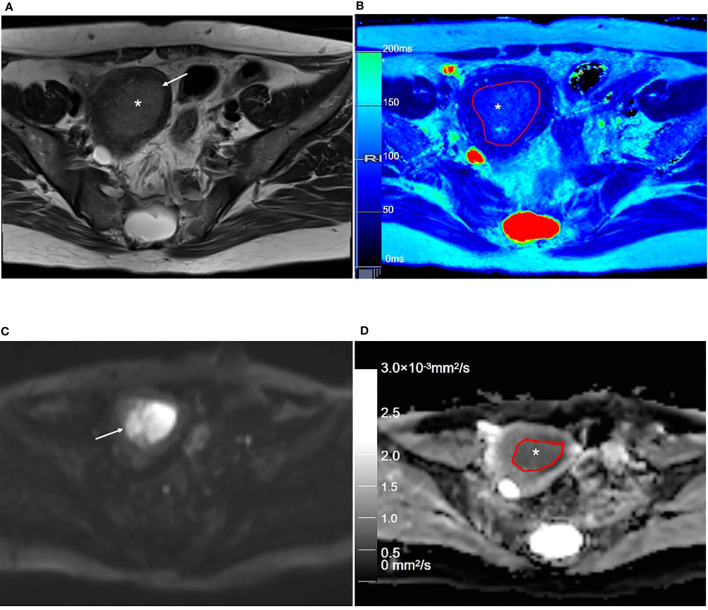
Representative T2WI and DWI and the corresponding axial T2 and ADC maps of the endometrium region of a 53-year-old patient with stage IA endometrial carcinoma. (A) Axial T2-weighted image shows there is an extensive, hyperintense mass lesion (*) confined to EMC in the uterine cavity. (B) Axial T2 map shows that the mass lesion (outlined in red, * in B) is slightly hyperintense. (C) Axial DWI (b = 1000 s/mm^2^) shows that the mass lesion (arrow) is hyperintense. (D) The axial ADC map shows that the mass lesion (outlined in red, * in D) is hypointense. T2WI, T2-weighted imaging; DWI, diffusion-weighted imaging; ADC, apparent diffusion coefficient; JZ, junctional zone.
